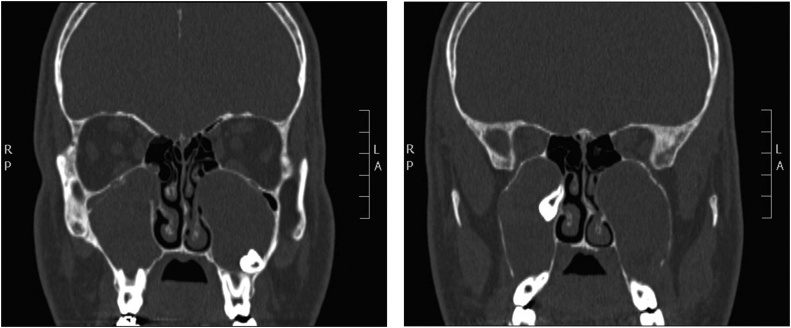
Coronal CT sinuses showing bilateral cystic lesions in the maxillary antra with expansion and remodeling of the roof, lateral and medial walls. The right cystic lesion displaces ectopic tooth superiorly and medially in the region of the expanded remodeled osteomeatal complex. The left ectopic tooth is impacted in the inferior portion of the lateral wall.
